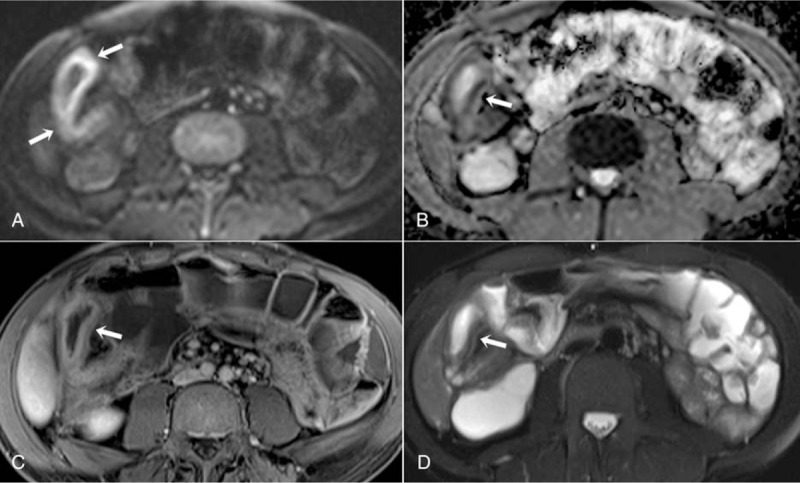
MR enterography findings of a 37-yr-old male patient show active inflammation in the right colon (arrows). Marked diffusion restriction is observed in the intestinal wall in the right colon on axial DWI (A) and ADC map (B) (scored 3 points). Mucosal hyperenhancement is observed on contrast-enhanced axial T1-weighted image (C), and wall thickness is observed on axial fat-suppressed T2-weighted image (D). Because contrast enhancement was less than the nearby vascular structure, it was scored as 2 points. ADC = apparent diffusion coefficient, DWI = diffusion weighted imaging, MR = magnetic resonance.
